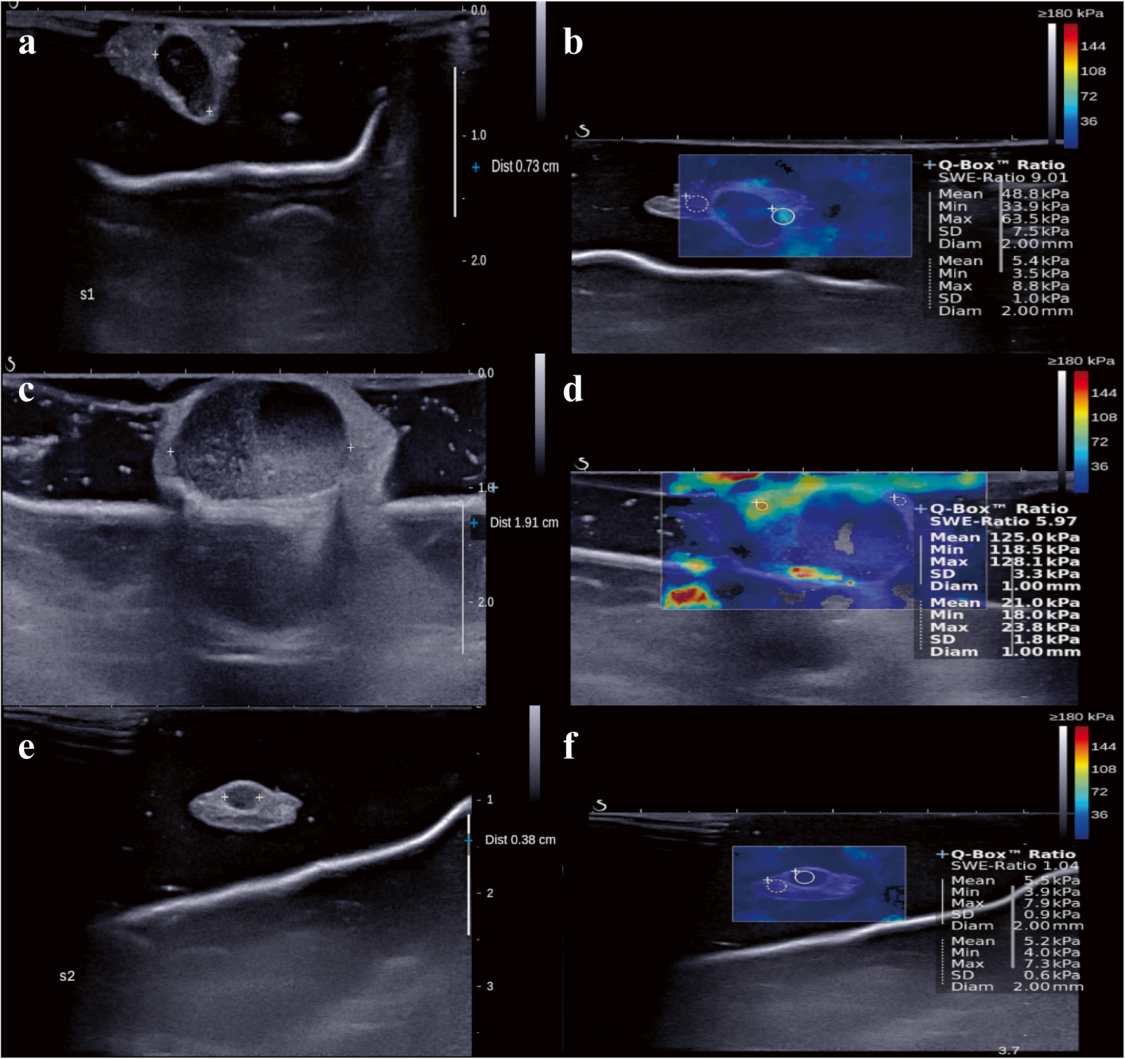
B-Mode ultrasound and shear-wave elastography (SWE). a B-Mode ultrasound showed 0.73-cm–sized excised sentinel lymph node with adjacent fat tissue. b The mean stiffness was measured by placing the 2-mm–sized region-of-interest (ROI) on the stiffest part of the excised sentinel lymph node (circle). The stiffness of the adjacent fat tissue was measured by placing another ROI on the surrounding fat tissue (dotted circle). Then, the elasticity ratio was calculated automatically. a–d B-Mode ultrasound and shear-wave elastography (SWE) images of patients with lymph node metastasis. e, f B-Mode ultrasound and shear-wave elastography (SWE) images of patient without lymph node metastasis
